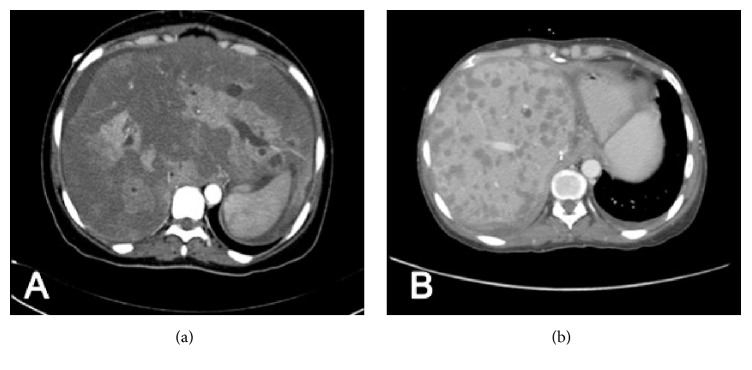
(a) Abdomen CT showing diffuse neoplastic infiltrative involvement of the entire liver with sparing of small patchy areas of the liver. (b) CT of the abdomen showing post-liver transplant recurrence with innumerable diffuse hypodense lesions in the liver.
